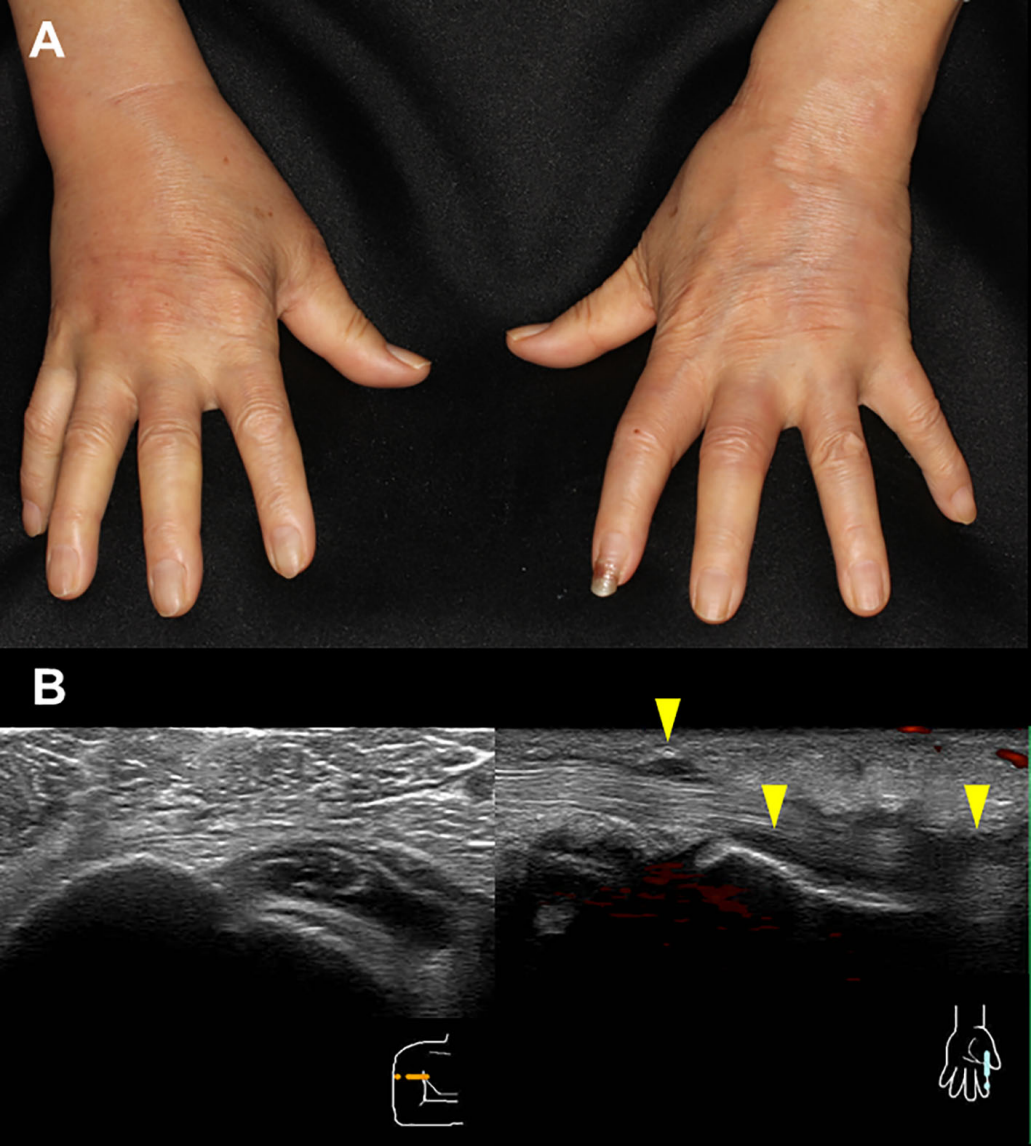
Edema of both fingers and the dorsa of both hands and erythema on the dorsal sides of the fingers (A). On ultrasonographic examination, fluid retention around the biceps, synovitis of the biceps, and synovitis of the joint synovium and tendon sheath synovium are observed (B).
